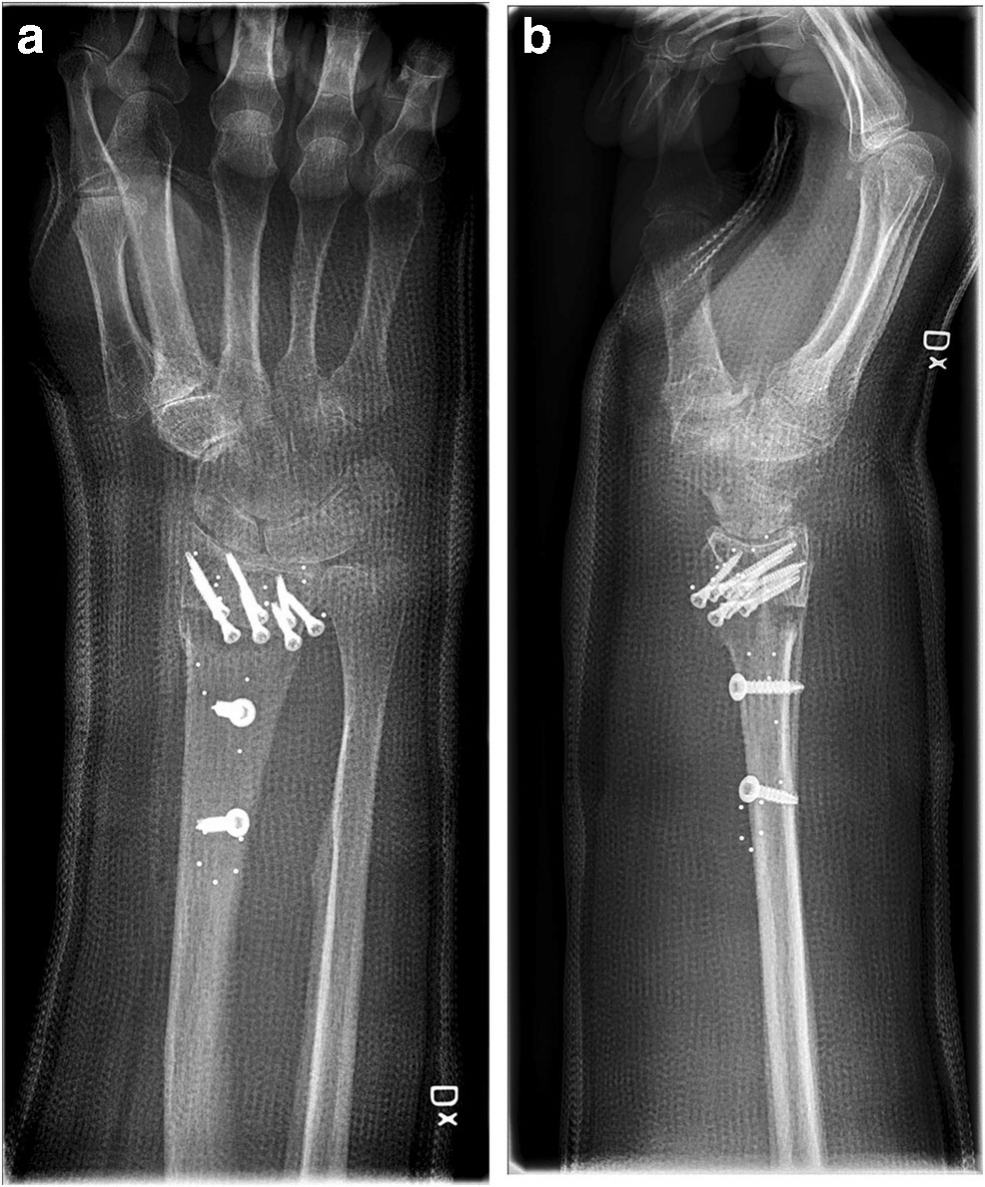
Post-operative radiographs after open wedge osteotomy. a AP view. b Sagittal view
